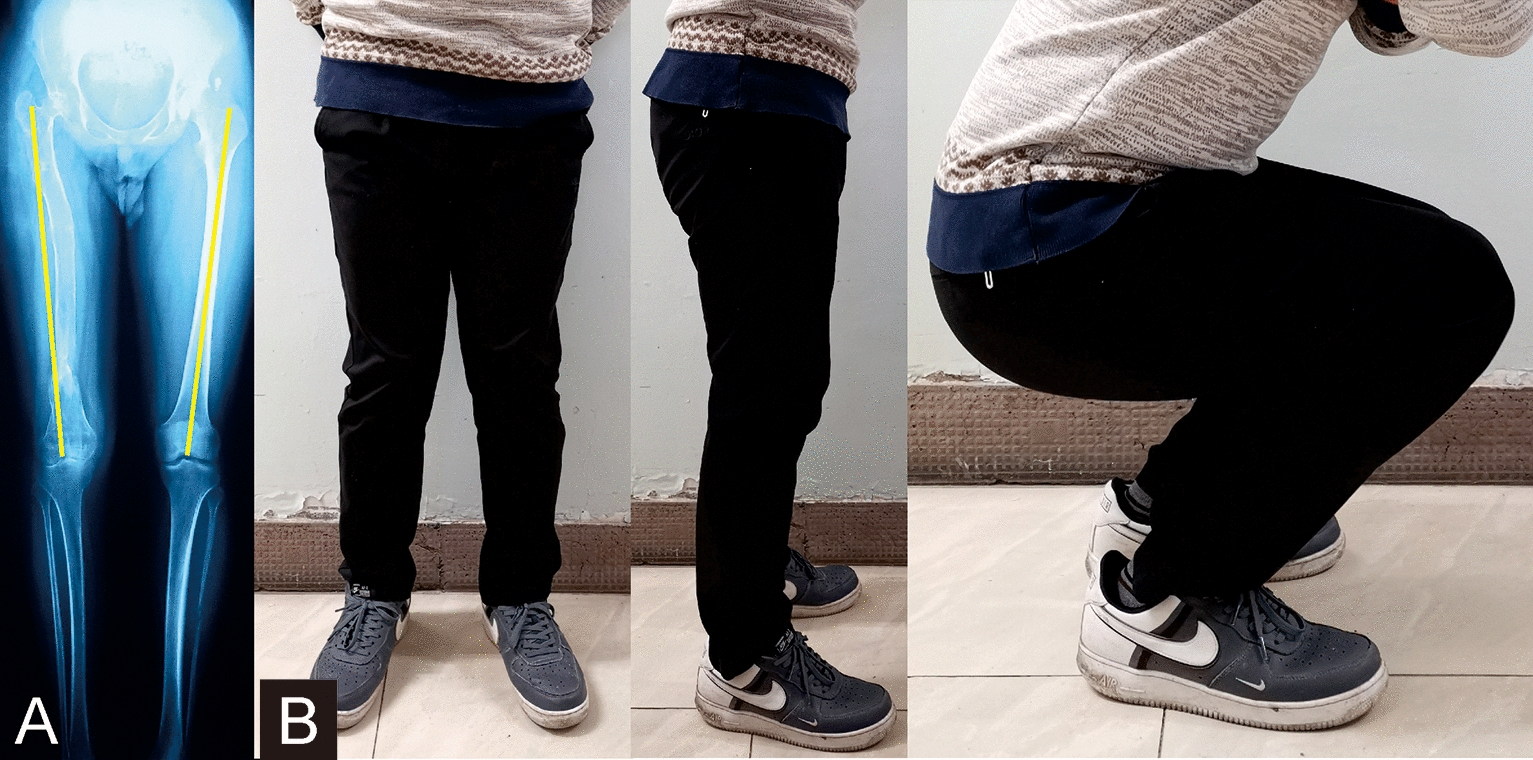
Follow-up images of the same patient (shown in Fig. 4) after removing the external fixator. a Radiograph 6 months later. b General images at the last visit 13 months later, showing the excellent functional results
